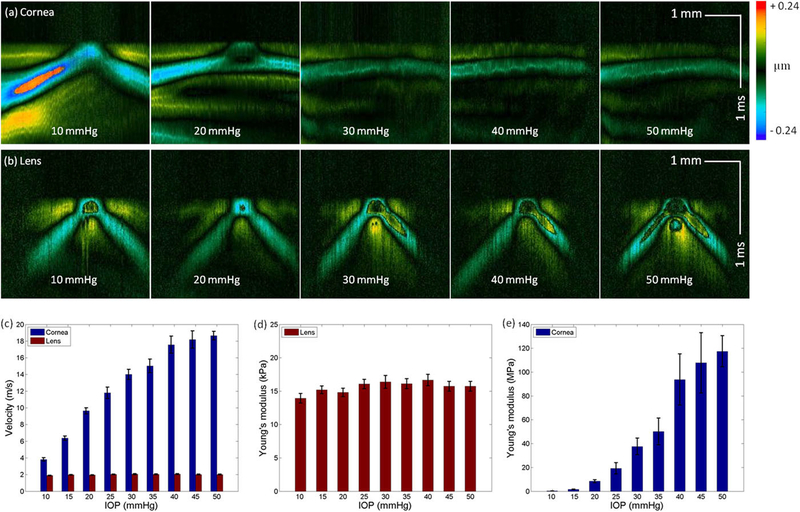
In vivo* IOP experiments using a 12-week rabbit. [(a) and (b)] Spatiotemporal Doppler OCT of the cornea and lens, respectively, at different IOPs. (c) Elastic wave velocities in the cornea and lens. (d) Young’s modulus of the cornea as a function of IOP. (e) Young’s modulus of the lens as a function of IOP.
